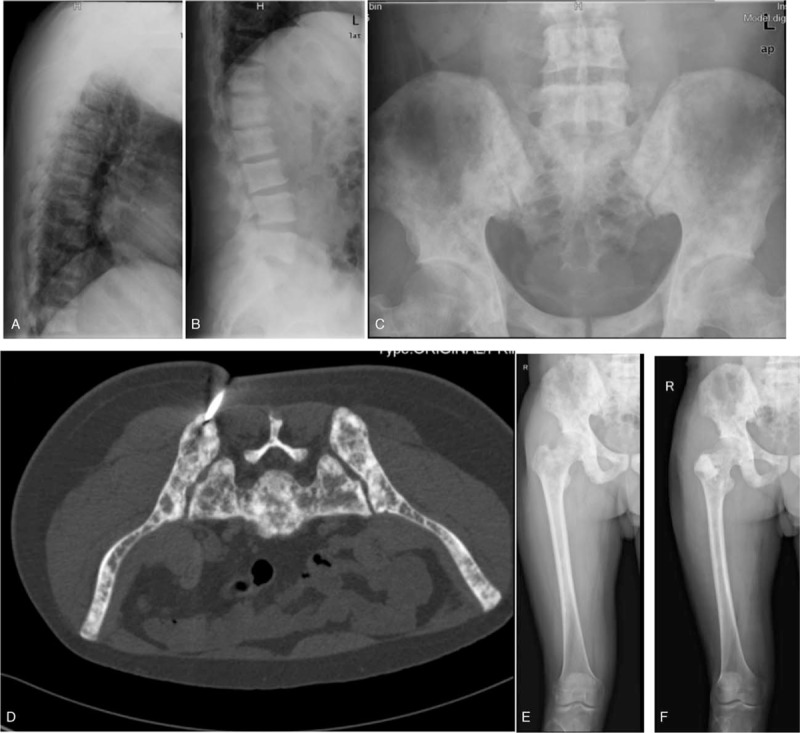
Bone imaging findings before Zometa therapy. (A and B) Lateral radiographs of the thoracic (A) and lumbar (B) vertebra showed flattening of the vertebral body, increase of bone density and widening of intervertebral space. (C) Pelvic plain radiographs showed multiple lesions with increased and decreased bone density. (D) CT-guided iliac bone biopsy, diffuse high and low mixed density lesions of the ilium and sacrum can be seen, with multiple bone destruction. (E) Prior to biopsy of the upper right femur, a general increase of bone density from the pelvis to the upper femur, with mixed high and low density. (F) After bone biopsy of the upper segment of the right femur, a cement filling shadow at the biopsy can be seen.
